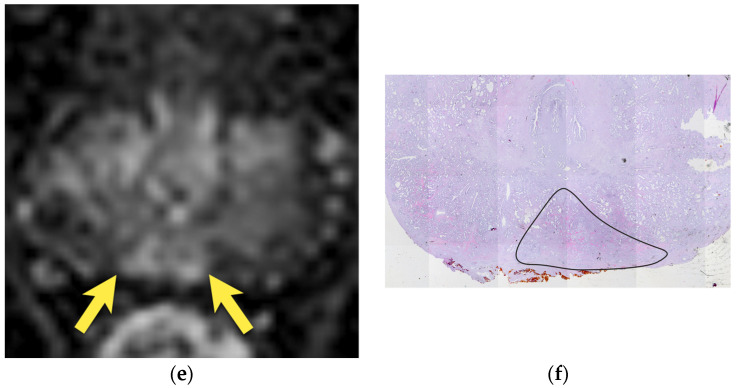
Prostate cancer in the median posterior gland in a 48-year-old patient with PSA 9.75 ng/mL (a) axial and coronal (b) T2-weighted images show the focal, ill-defined area of low signal intensity (arrows), with marked diffusion restriction on (c) DWI b 2000 image (arrows) and (d) ADC map (arrows); and early enhancement (arrows) (e). The lesion was assessed as PI-RADS 4. (f) After radical prostatectomy, PCa GS 4 + 3 was diagnosed in this location (area outlined with black continuous line).
